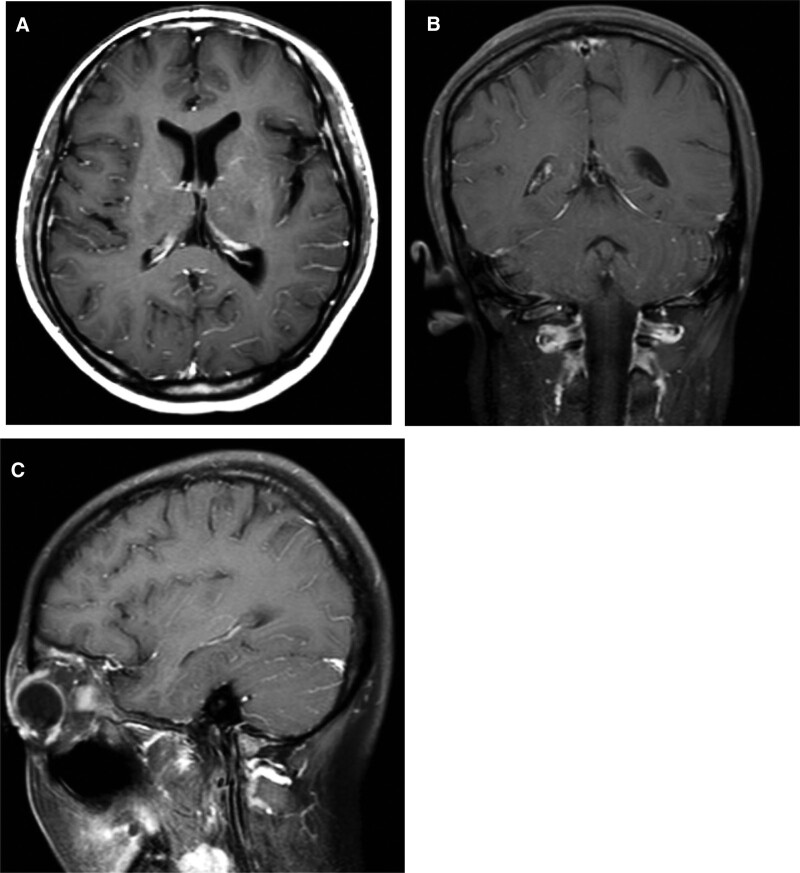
A, B and C show the MRI axial, coronal and sagittal enhancement images on the 10th day of admission, with multiple linear enhancement shadows in the cerebral and cerebellar sulci with smooth margins. MRI = magnetic resonance imaging.
